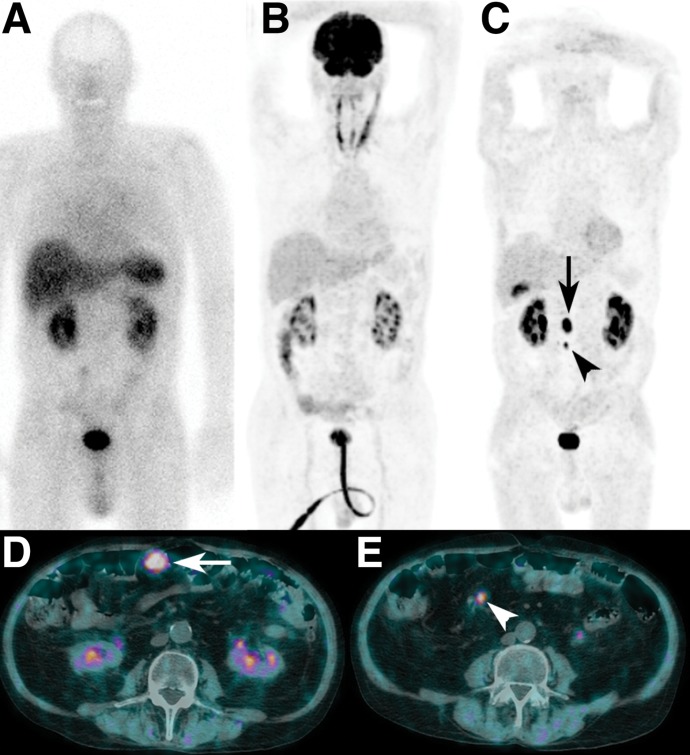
(A) ^111^In-octreotide scintigraphy and (B) ^18^FDG-PET did not reveal any pathological tracer uptake. (C) ^18^F-DOPA PET-CT allowed to locate a primary jejunal tumour (D) (arrow) and revealed metastatic lymph node(s) (E) (arrowhead).
